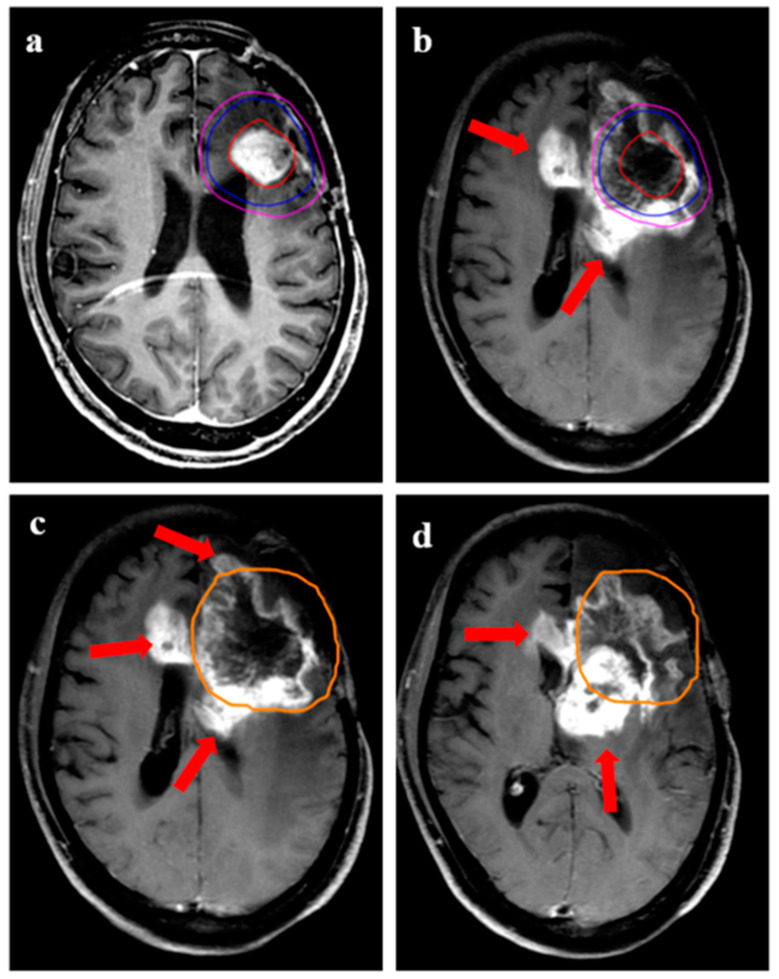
Demonstration of marginal recurrence following re-irradiation for a 43-year female with transformed high-grade glioma. (a) shows the target volumes (GTV: red, CTV: blue; PTV: magenta) on T1w contrast MRI. The GTV was the enhancing disease, with CTV drawn as an expansion of 10 mm around the enhancing disease. (b,c) demonstrates recurrent disease (arrows) with respect to target volumes and 95% isodose line (orange), 1. (d) is a representation from lower axial slices showing the growth of recurrent disease further beyond the 95% isodose line.
